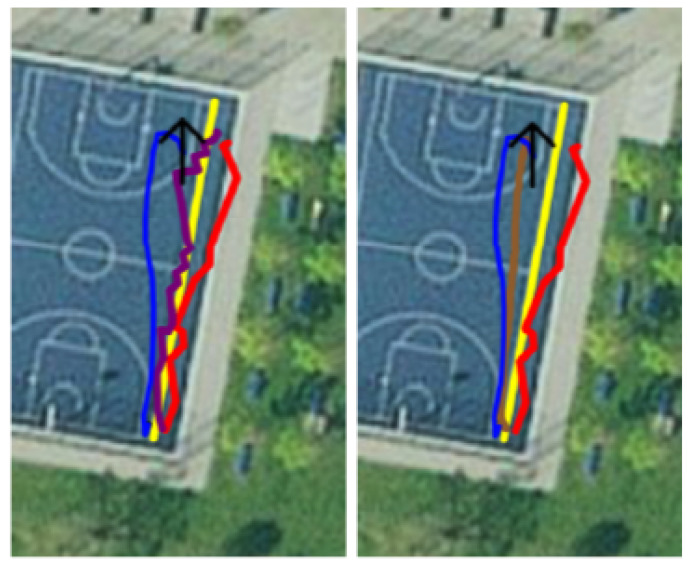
Jittering signal of KF Vel CT (purple) and smooth signal of KF CT (brown).
